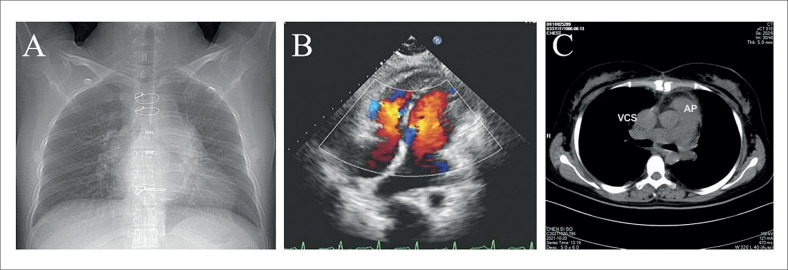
Radiografia de tórax pós-operatória (A), ultrassonografia cardíaca (B) e tomografia computadorizada (C) revelaram alívio da compressão da via de saída do ventrículo direito. VCS: veia cava superior; AP: artéria pulmonar.
